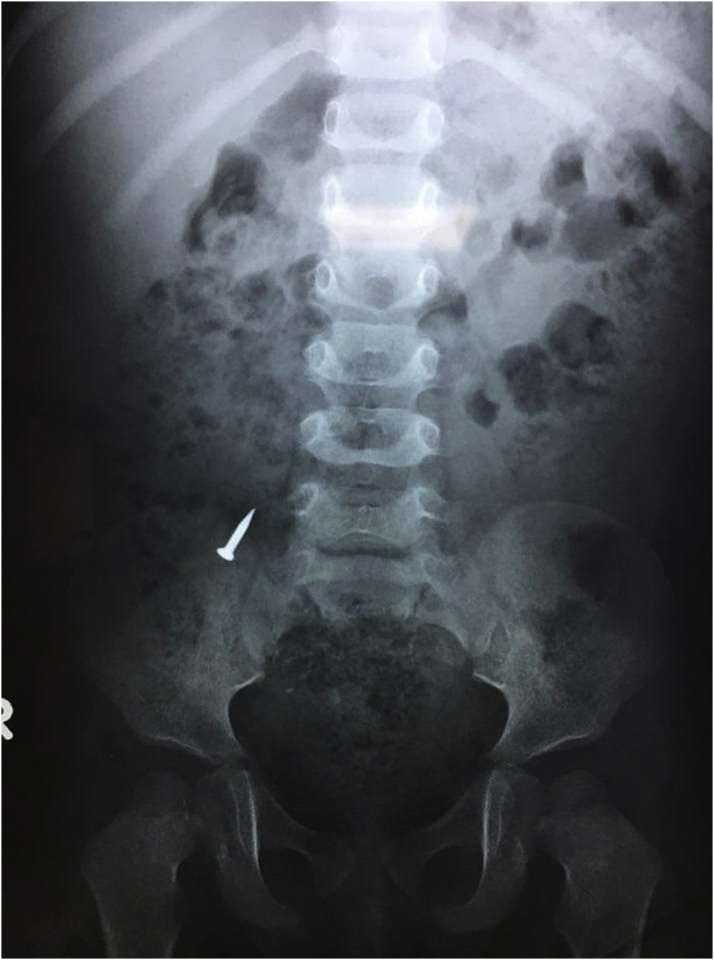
Plain abdominal X-ray in erect position showing the metallic nail in the region of the right iliac fossa.
